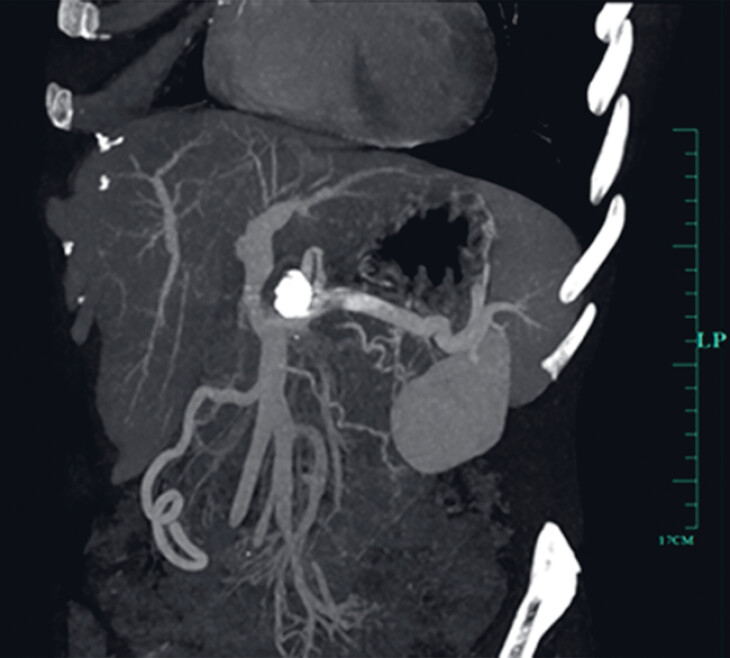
Gastric artery CTA after the EUS second intervention showed the GDAP to be completely embolized.
